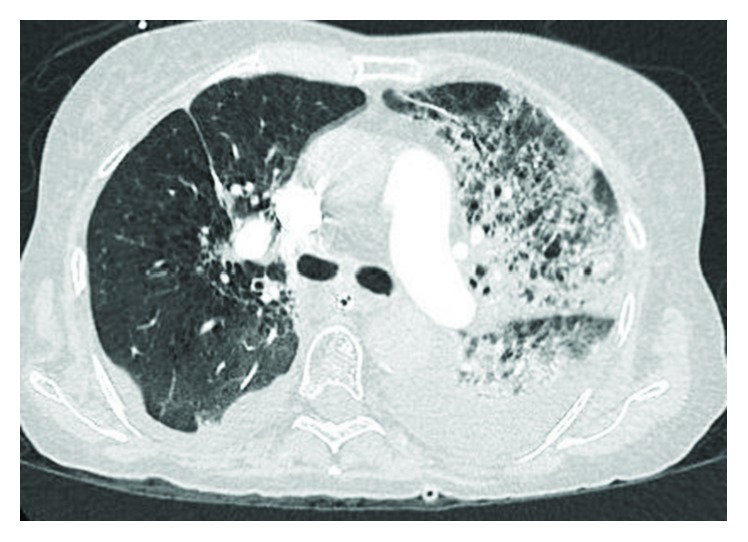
Representative axial CT for PE image of our patient upon admission to the intensive care unit showing dense airspace opacities involving predominantly the left upper lobe and small pleural effusion.
